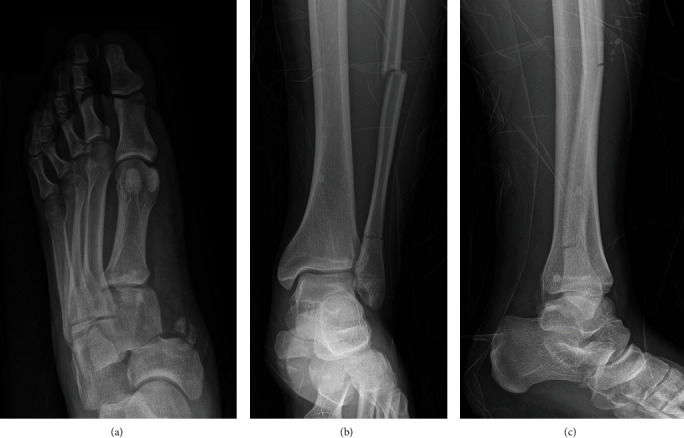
(a) Anteroposterior X-rays of the left foot show a dislocation through the naviculocuneiform joint with bony avulsions. (b) Frontal and (c) lateral X-rays of the ankle show a bifocal fracture of the fibula.
